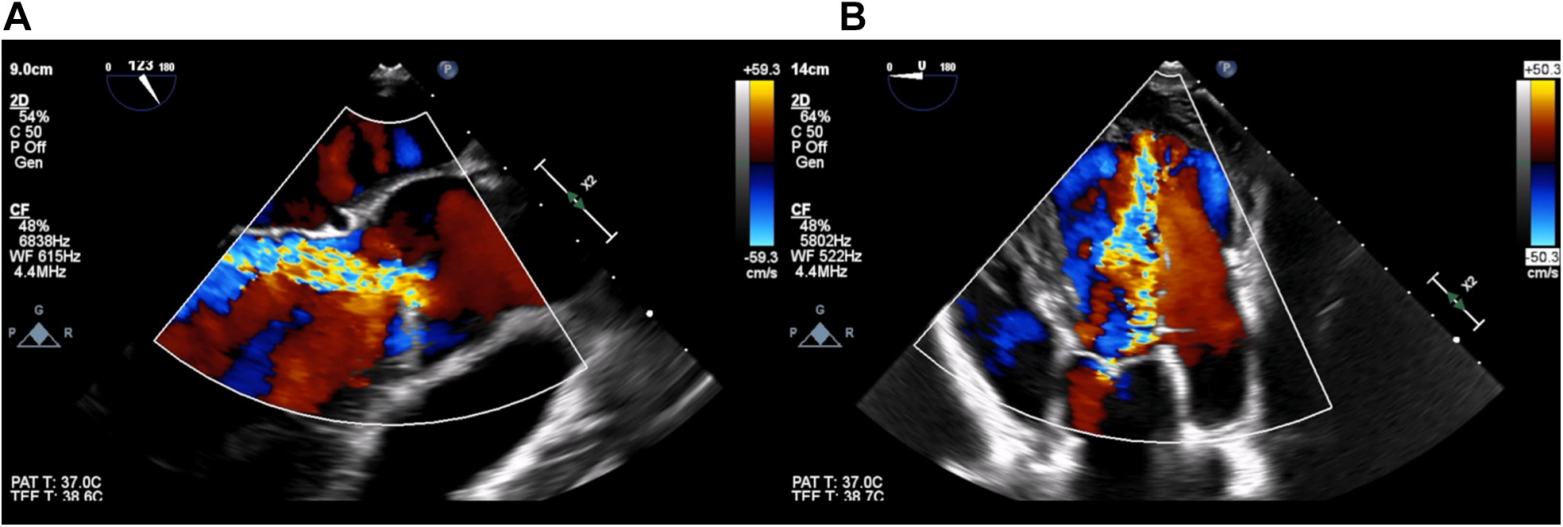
Transesophageal Echocardiography (A) Midesophageal long-axis view and (B) transgastric view showing severe aortic regurgitation. CF = color flow; WF = frequency sound waves; other abbreviations as in Figure 1.
